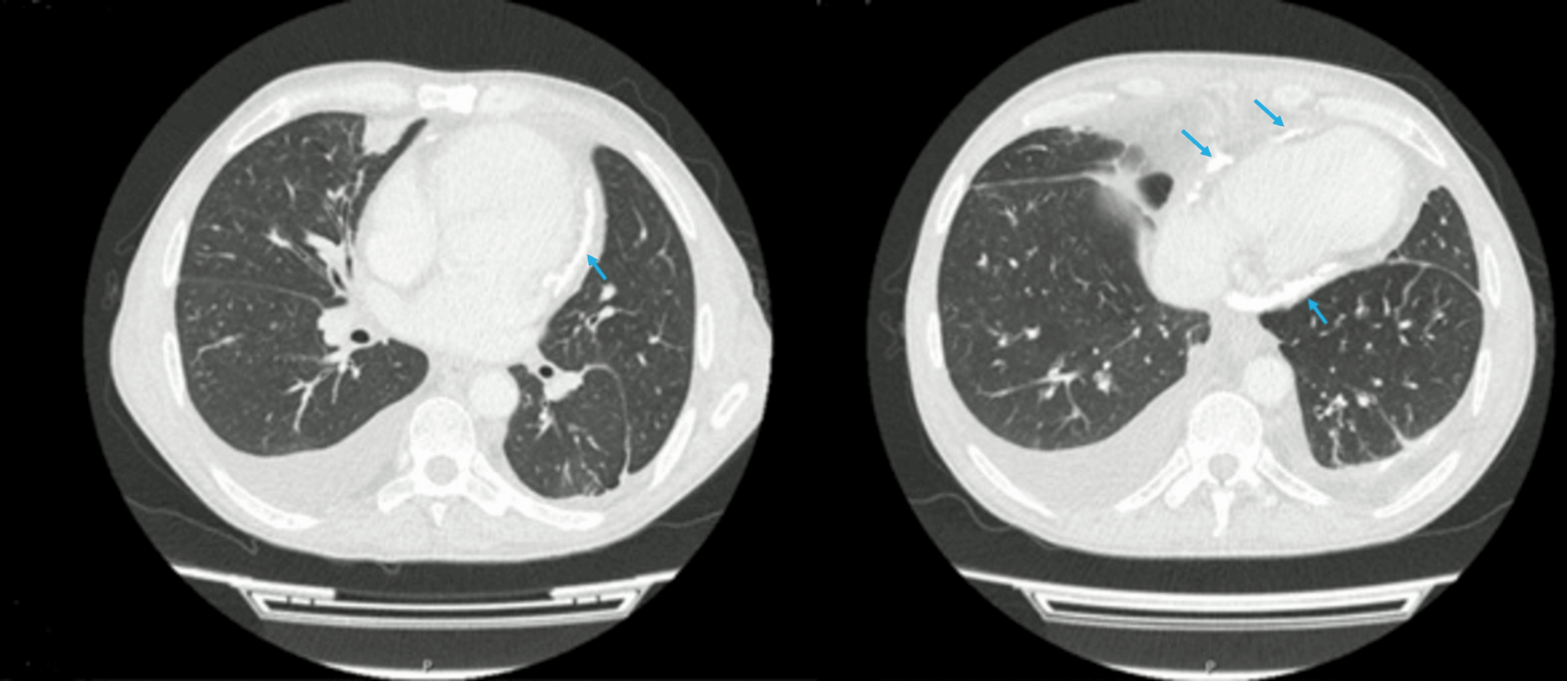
Chest CT scan of patient two demonstrating extensive pericardial calcifications (blue arrows), in particular in the anterior and inferior walls of the pericardium.
